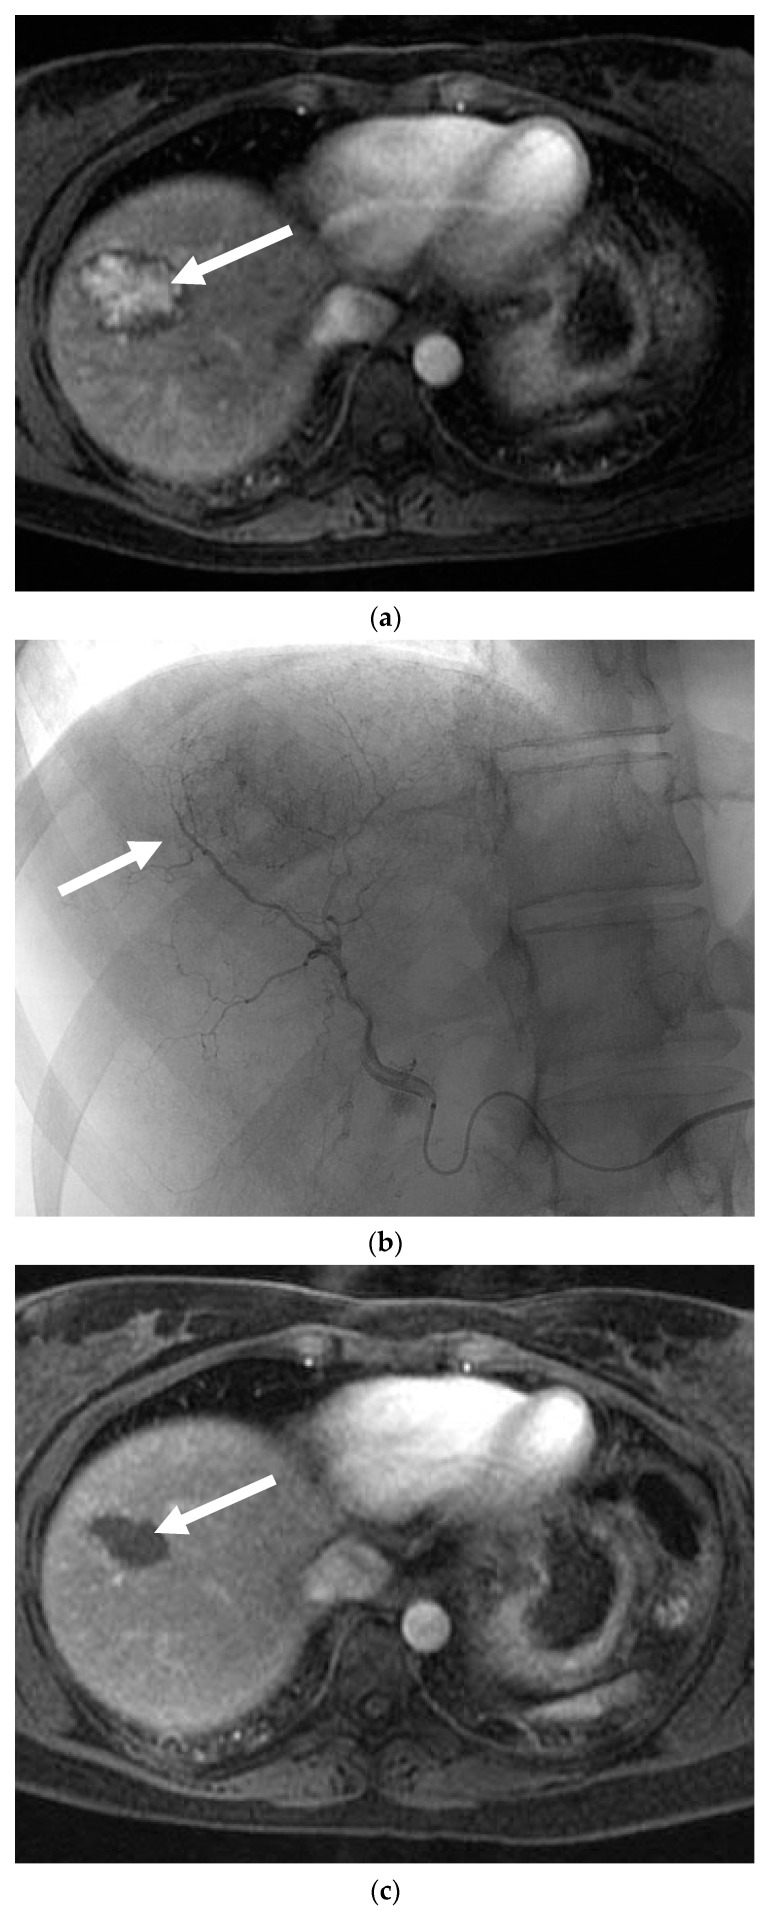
Hepatic adenoma following embolization. (a) MRI with gadolinium shows an enhancing 4.3 cm lesion in segment 8 (solid white arrow). This was symptomatic and had increased in size over the past year. (b) Selective injection of the right hepatic artery demonstrating the hypervascular hepatic adenoma (solid white arrow). This was treated with 40–100 µm and 100–300 µm tris-acryl gelatin microspheres. (c) Three months following embolization, MRI with contrast shows complete necrosis of the adenoma with interval decrease in size (solid white arrow).
